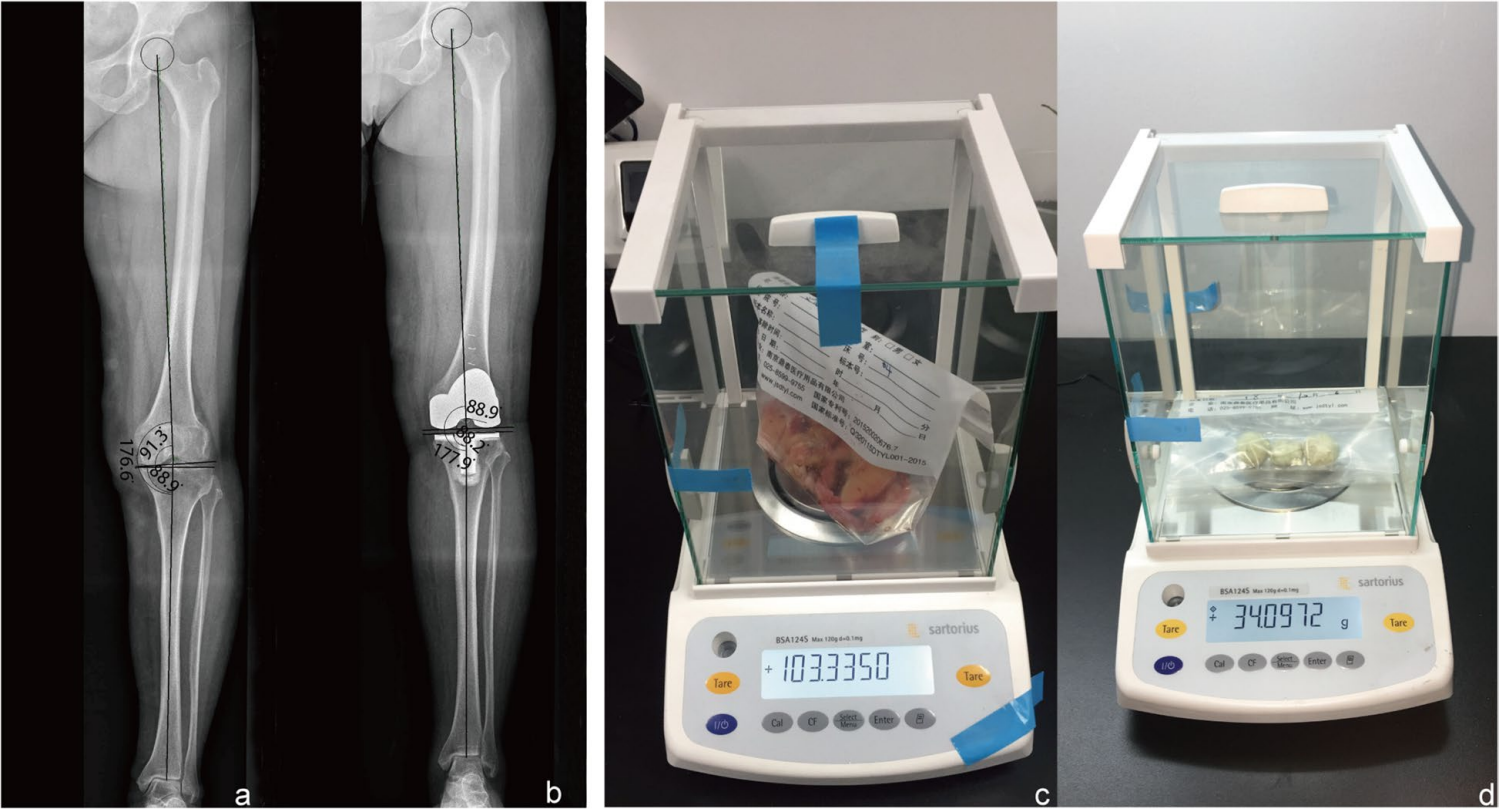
X-ray measurement of knee joint as well as weight measurement of excised knee tissue and unused bone cement. a HKA angle measurement before TKA; b HKA angle measurement after TKA; c weight of excised knee tissue; d weight of unused bone cement
